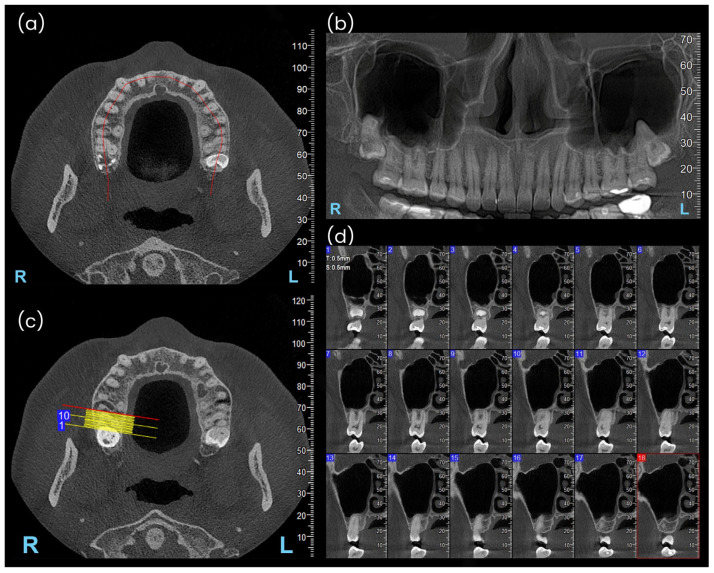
Radiographic data processing algorithm: (a) Reference axial image of CBCT used for curved panoramic reformation (red curved line); (b) Panoramic reformatted image; (c) Setting up buccolingual sections for tooth 17 on the reference axial image; (d) Set of serial cross-sectional images perpendicular to the curve of the dental arch and to tooth 17.
